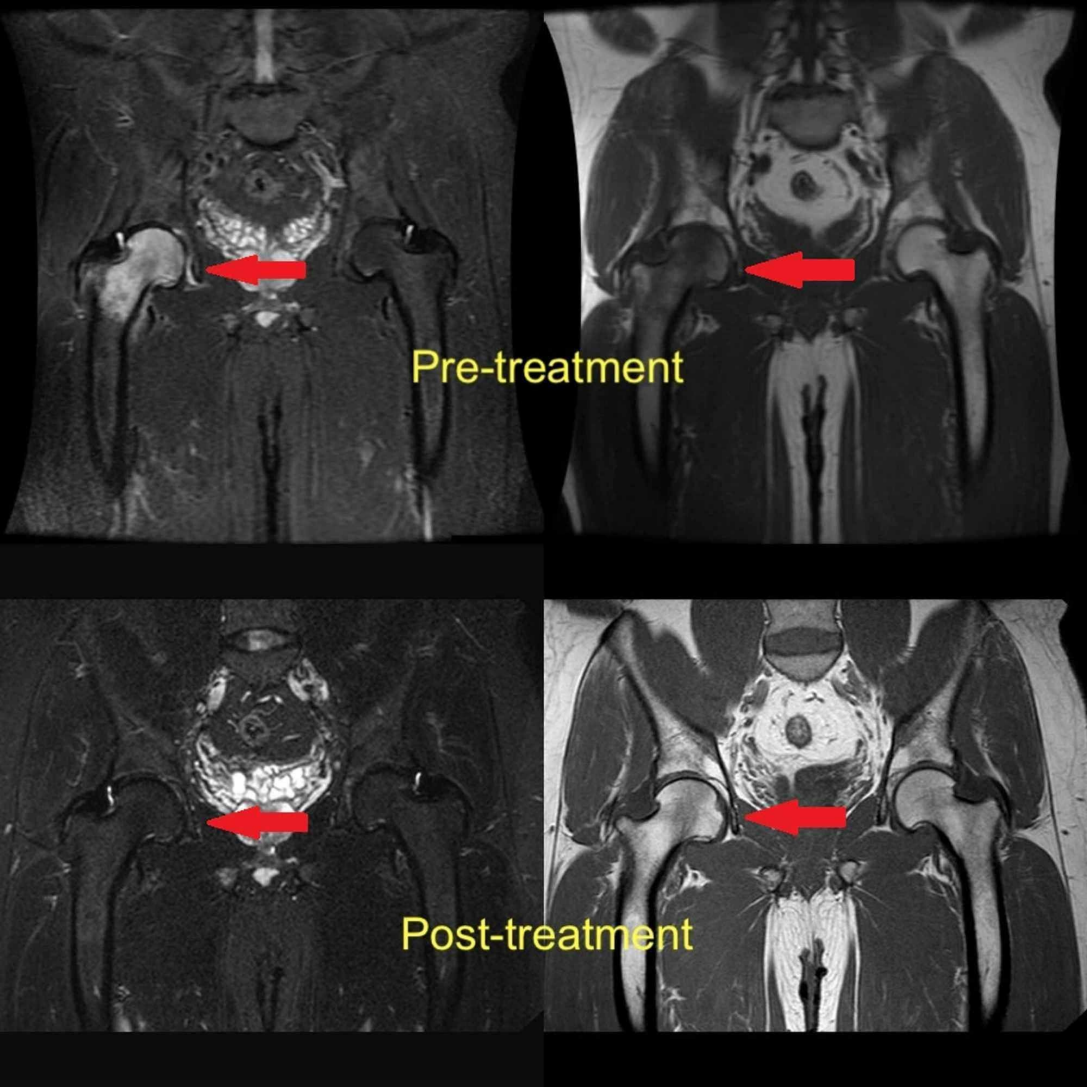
MRI appearance of bone marrow edema of the proximal femur (arrows) MRI: magnetic resonance imaging
